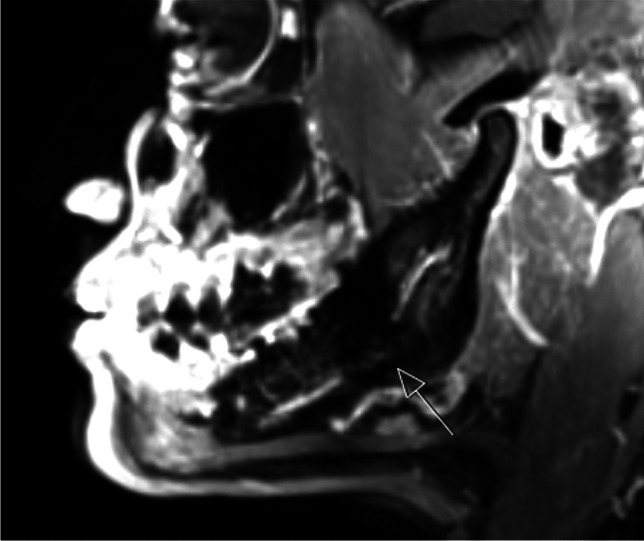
Magnetic resonance imaging of the right lower jaw from the patient depicted in Fig. 1, shown in a sagittal section. The nerve, presented as a light-shaded hyperintense structure, is clearly discernible within the darker, hypointense bone structure. The arrow highlights a conspicuous interruption in the structure of the inferior alveolar nerve precisely at the location of the prior wisdom tooth osteotomy. This observation significantly aids in the diagnosis of nerve damage
